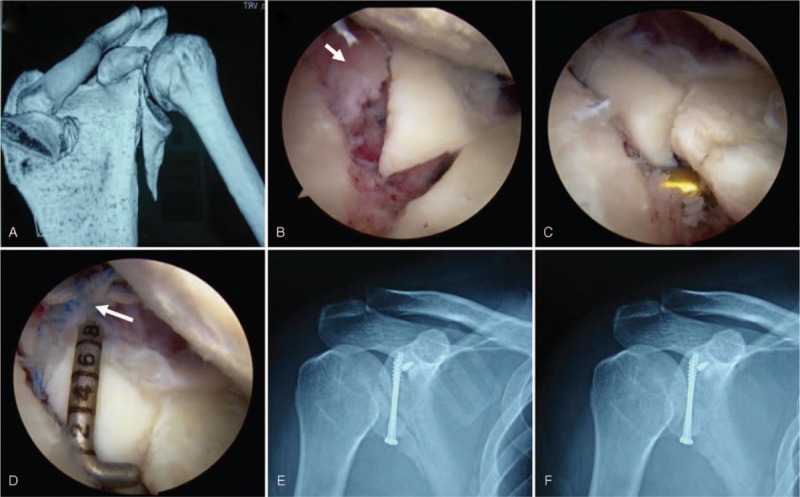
A, A 37-year-old man left suffered glenoid fracture after a traffic accident. A CT-3D image shows a displaced bone fragment. B, An arthroscopic image showing a small, displaced bone fragment near the fracture line (short arrow). C, Arthroscopic reduction of the bone fragments using a probe. D, The small bone fragment was fastened and fixed using the suture (long arrow). At postoperative 1 day (E) and 12 weeks (F), bone union was achieved. The X-ray films show the position of the cannulated screws and the suture anchor.
